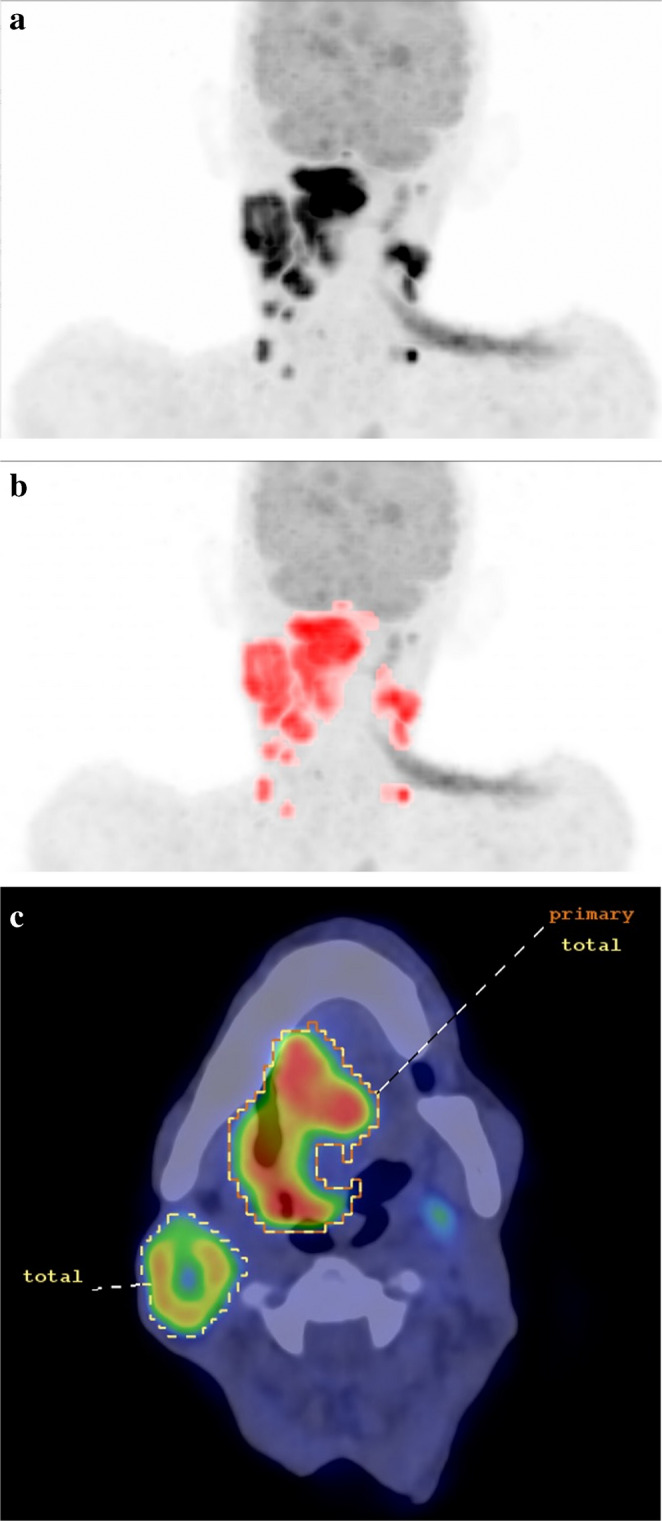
Tumor segmentation procedure. a) original three-dimensional PET maximum intensity projection (MIP) image of the head and neck, intensive uptake of the meso-hypopharyngeal squamous cell carcinoma and the bilateral neck lymph node metastases; b) automatic segmentation of the total tumor volume with SUV low threshold of 2.5 g/ml (volumes of interest in red), this method was kept as reference, since it could not exclude every region with physiologic or inflammatory uptake close to the tumor; c-d) semi-automatic segmentation of the primary tumor (orange) and total tumor volume (yellow, primary and metastatic nodes together) using a SUV low threshold of 2.5 g/ml and manual correction if needed according to the anatomical landmarks on CT
